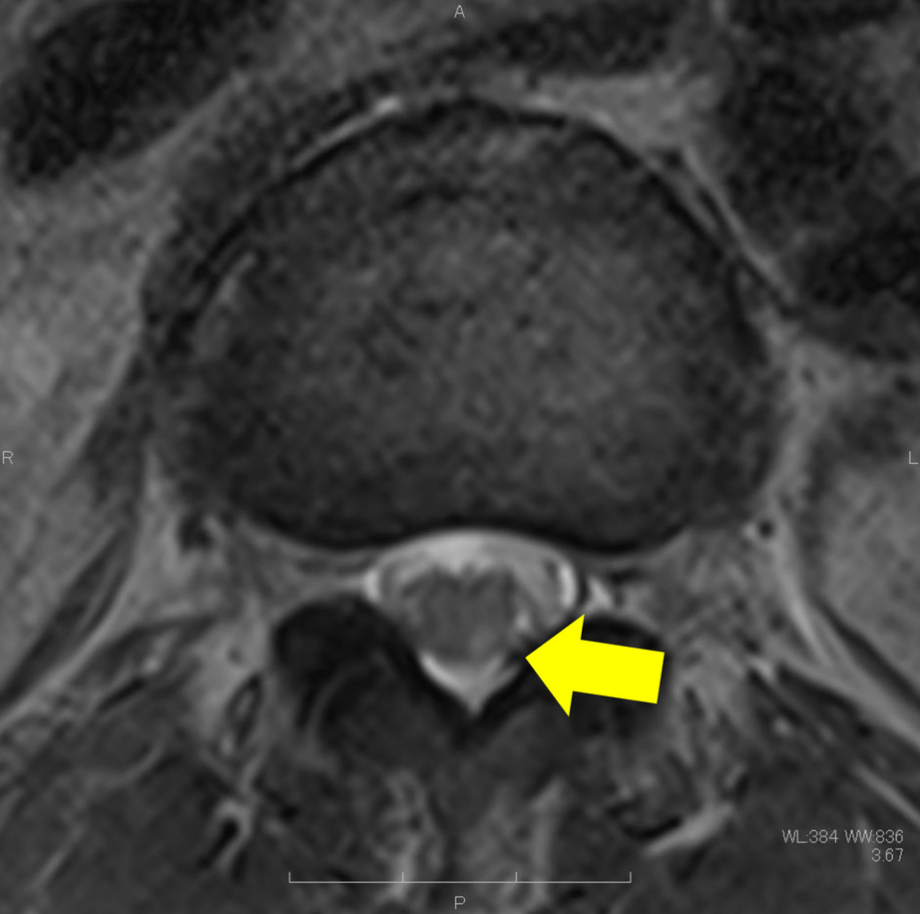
T2-weighted magnetic resonance imaging (MRI) at the L3 level showed a high signal, indicating ischemia, in the left medulla of the lumbar spinal cord (yellow arrow).
